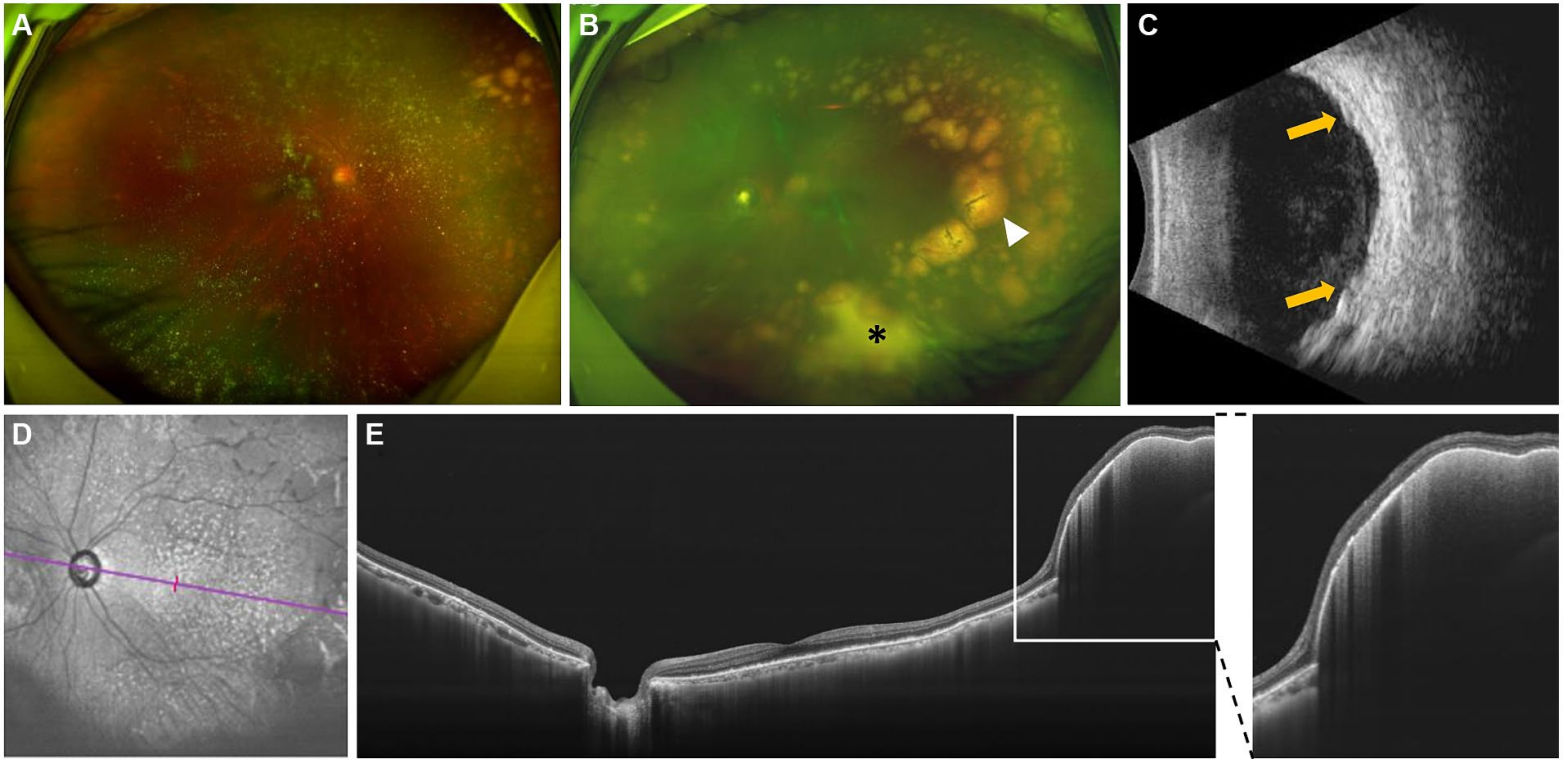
Fundus photographs, B-scan ultrasonography and swept-source Optical Coherence Tomography (SS-OCT) examinations at initial presentation. (A) Asteroid hyalosis and several subretinal yellow deposits were detected in the nasal retina of the right eye. (B) Moderate vitreous opacity, multiple subretinal yellow nodular lesions with varying sizes, streaky pigmentation (white arrowhead) and white exudation (asterisk) on the surface of several yellow subretinal nodules were detected in the left eye. (C) B-scan ultrasonography showed clusters of moderately or highly condensed punctate echoes in vitreous cavity, and irregular hypoechoic lesions (yellow arrows) under the retina of the left eye. (D,E) Infrared image and SS-OCT images with scanning length of 24 mm of the left eye. Homogenous hyperreflective lesions in the sub-RPE space were detected in the peripheral retina. An enlarged inset is provided on the right.
